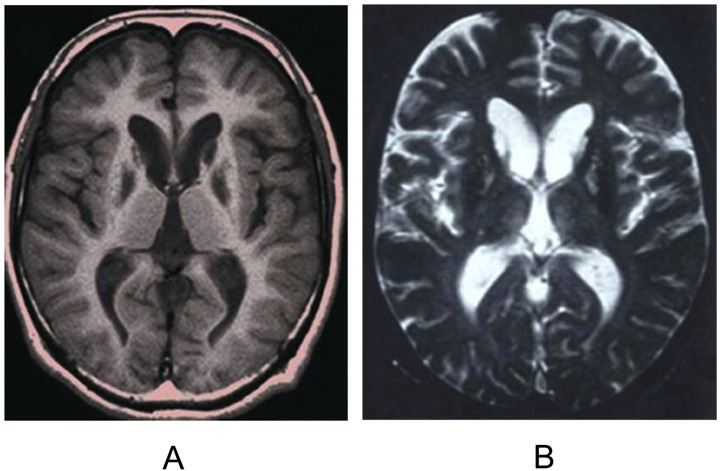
Brain MRI in Neuroferritinopathy. Brain MR images of a Japanese man carrying the *FTL1* c469_484dup mutation. Axial section at the level of the basal ganglia in the patient at 35 years of age. A A T1-weighted image (TR 400 msec/ TE 14 msec) shows symmetrical hypointense signals in the head of the caudate nucleus and globus pallidus. B A T2-weighted image (TR 800 msec/TE 30 msec) shows hypointense changes in the lenticular nucleus. Hyperintense signals can be observed in the putamen and the head of the caudate nucleus. Reproduced from Ohta E, Takiyama Y. MRI findings in neuroferritinopathy. Neurol Res Int. 2012;2012:197438.
